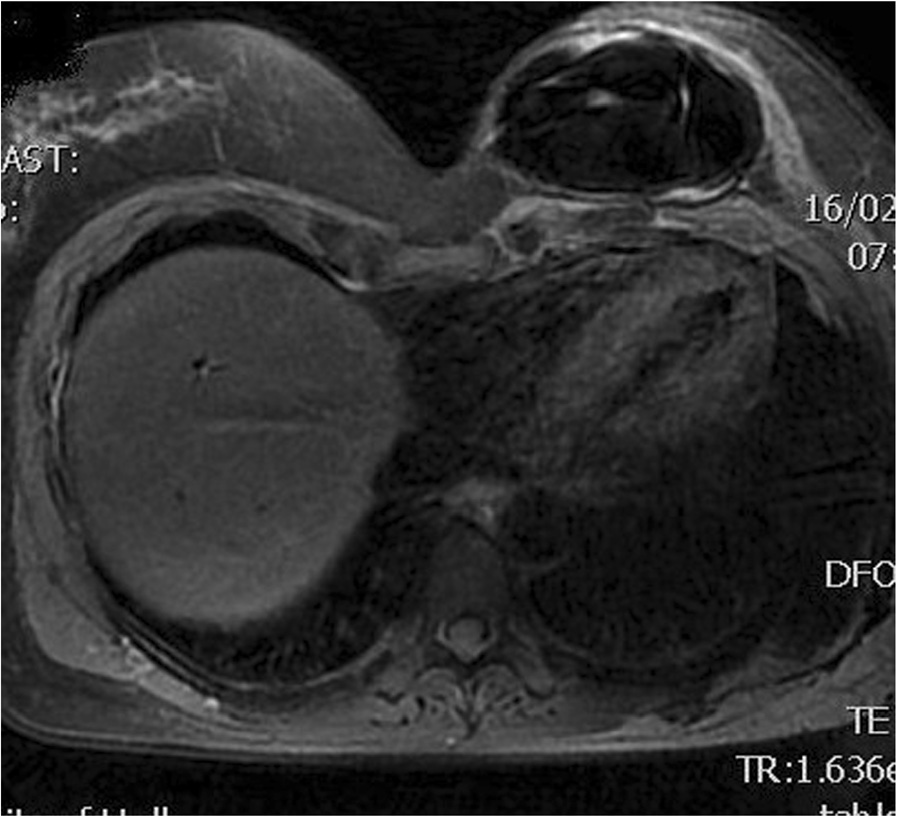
Magnetic resonance imaging (MRI) showing capsular contracture of left breast implant and posterior latissimus dorsi (LD) mass.
